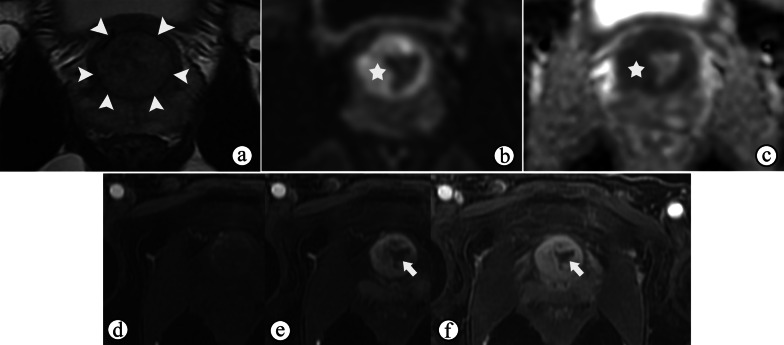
Prostate stromal tumor of uncertain malignant potential in a 33-year-old man with a normal serum PSA level of 1.080 ng/mL. a Axial T2WI shows a 2.7-cm nodule with heterogeneous signal in the TZ of the prostate surrounded by a capsule-like hypointense rim (arrowhead). b, c DWI and ADC maps show remarkably restricted diffusion of most of the nodule (☆). d–f Axial DCE imaging at 30 s (d), 60 s (e) and 120 s (f) after contrast injection shows early and gradual enhancement of the nodule containing non-enhanced areas inside the tissue (arrow)
